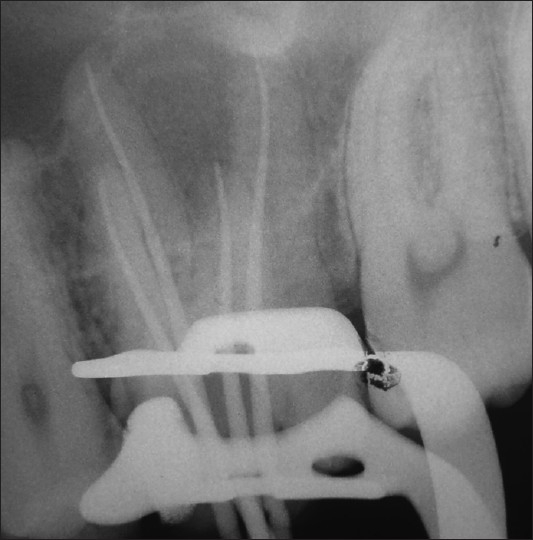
Master cone radiograph of 26
